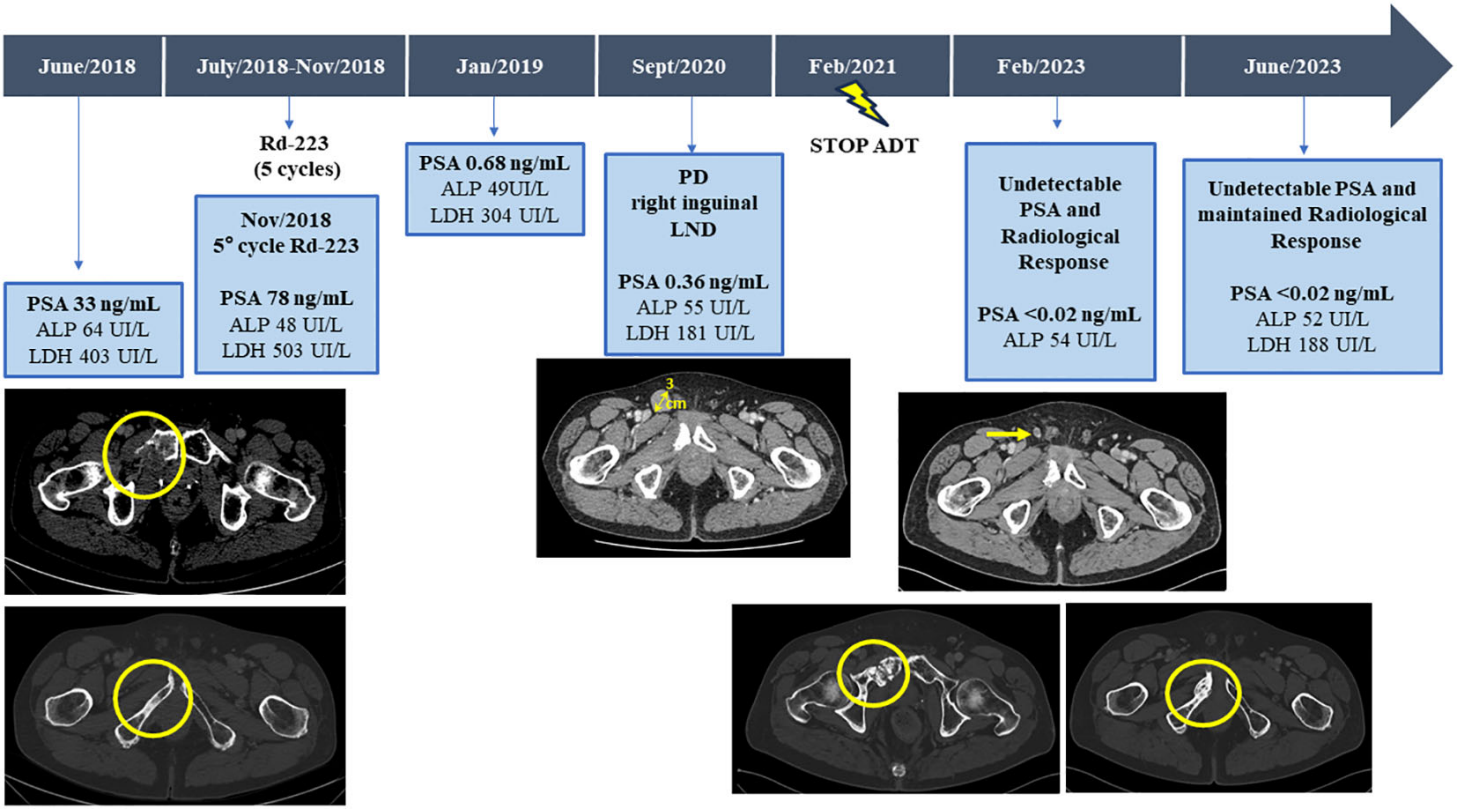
Serum bone biomarkers values (ALP, LDH, and PSA) and CT scans before and after third line with Radium-223 treatment, throughout the disease course. ALP, alkaline phosphatase; LDH, lactic acid dehydrogenase; PSA, Prostate-specific antigen; CT, computed tomography.
